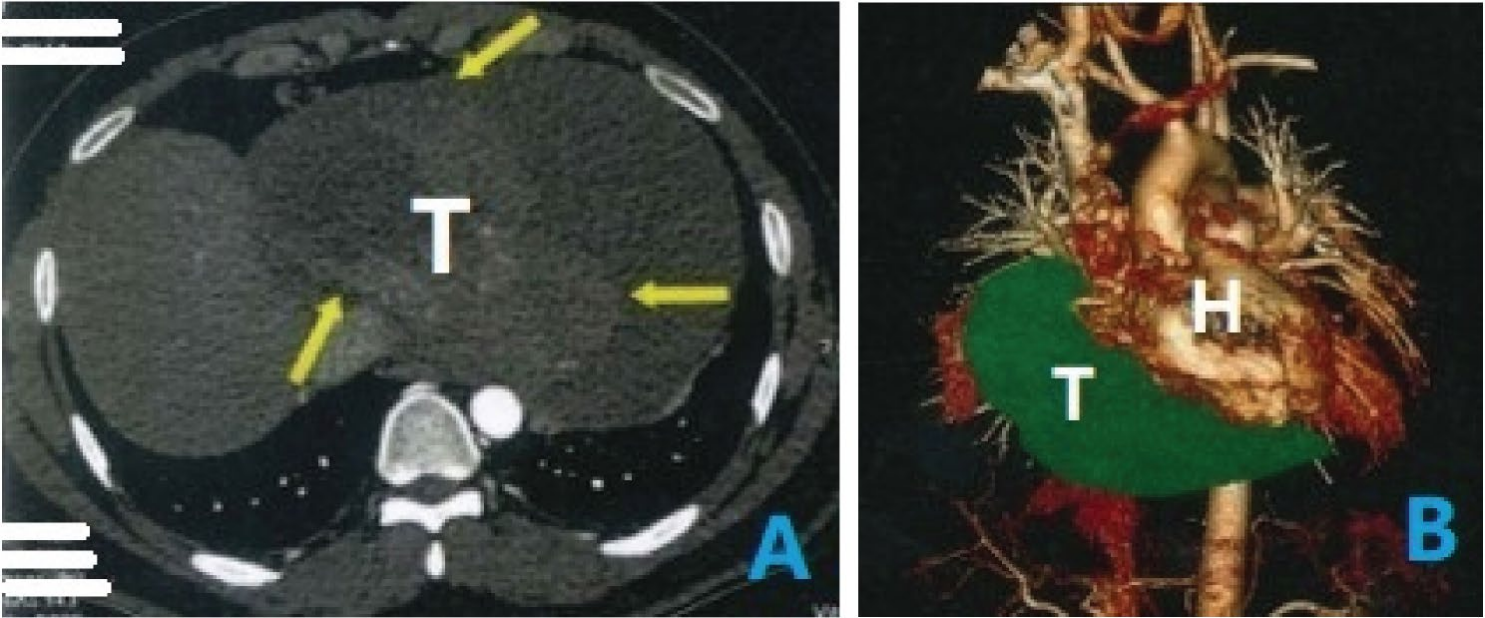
Plain computed tomography scan (A) and three-dimensional computed tomography scan (B) show a giant triangular pyramid mass adjacent to the ventricular diaphragmatic side of the heart. T: tumor; H: heart
